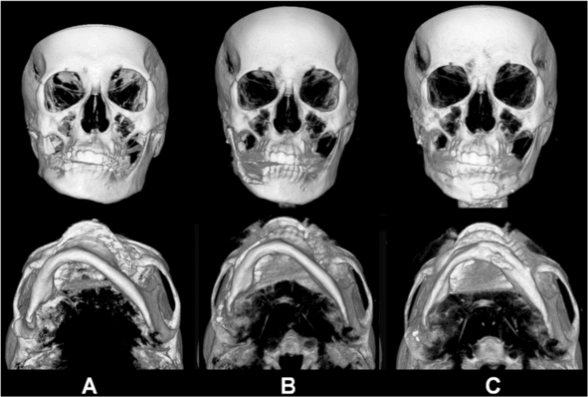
Three-dimensional computed tomography images taken at each successive surgical step. a Preoperative. b After gap arthroplasty and distraction osteogenesis. c After mandibular contouring surgery to correct residual asymmetry of the mandible
